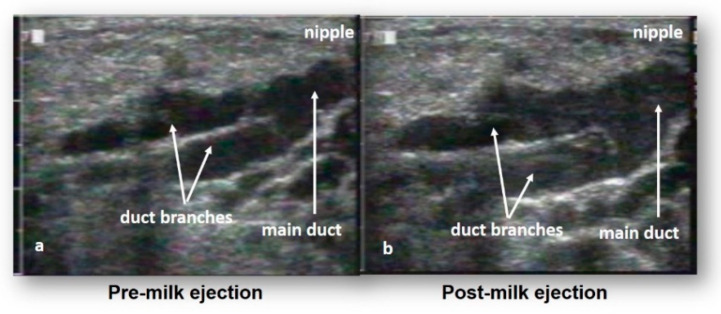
Ultrasound images of milk ducts pre-milk ejection (a) and post-milk ejection (b). Milk ducts are imaged as black (anechoic). Post milk ejection the ducts are expanded with breast milk (Geddes et al., unpublished).
